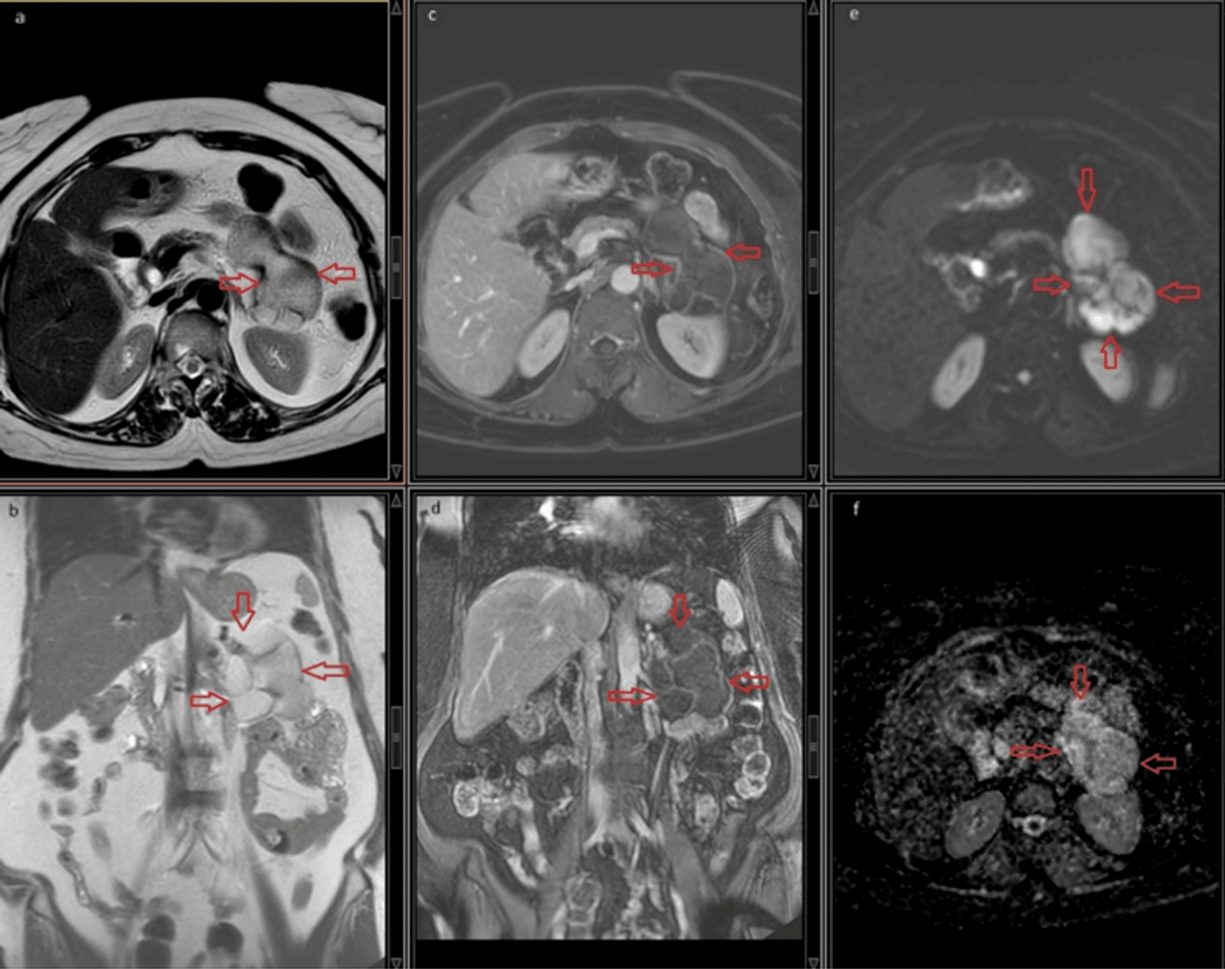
(a,b) Intracystic fluid as evidenced by high intensity on T2WI in the axial-coronal plane (arrows). (c,d) Significant multilobular formation and slight thickening of contrast enhancement along the septal wall (arrows). (e,f) Absence of any significant restriction or solid component (arrows). T2WI: T2-weighted imaging
